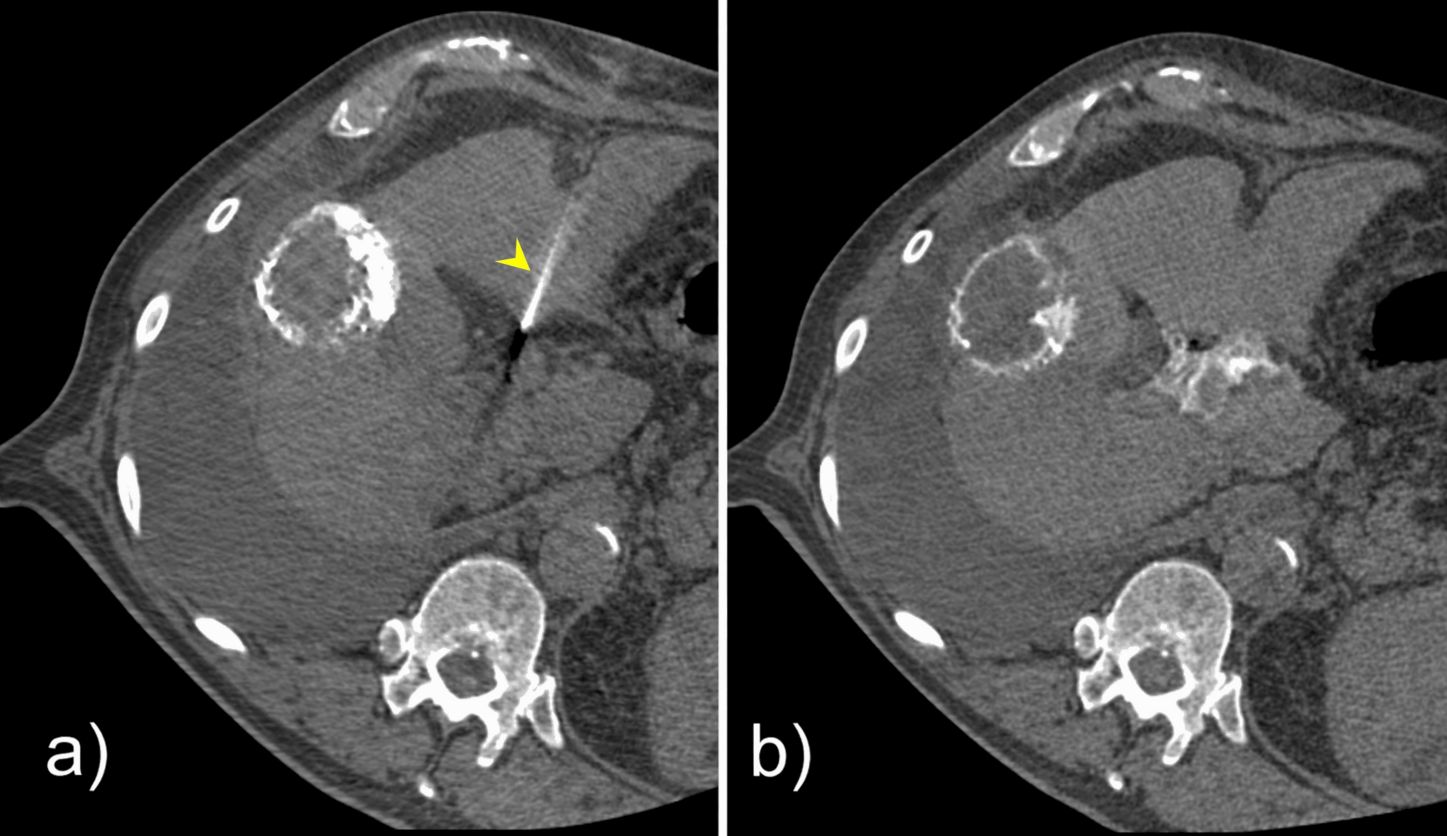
Computed tomography (CT) images of the patient (A) Axial computed tomography (CT) image with 3-mm slice thickness and 1-mm reconstruction interval demonstrating a 22-gauge needle (yellow arrowhead) positioned within the periportal space at the porta hepatis. (B) Repeat axial CT image obtained after administration of 5 mL of iodinated contrast (Visipaque® 320), demonstrating periportal contrast distribution without evidence of intravascular opacification, confirming appropriate needle positioning.
